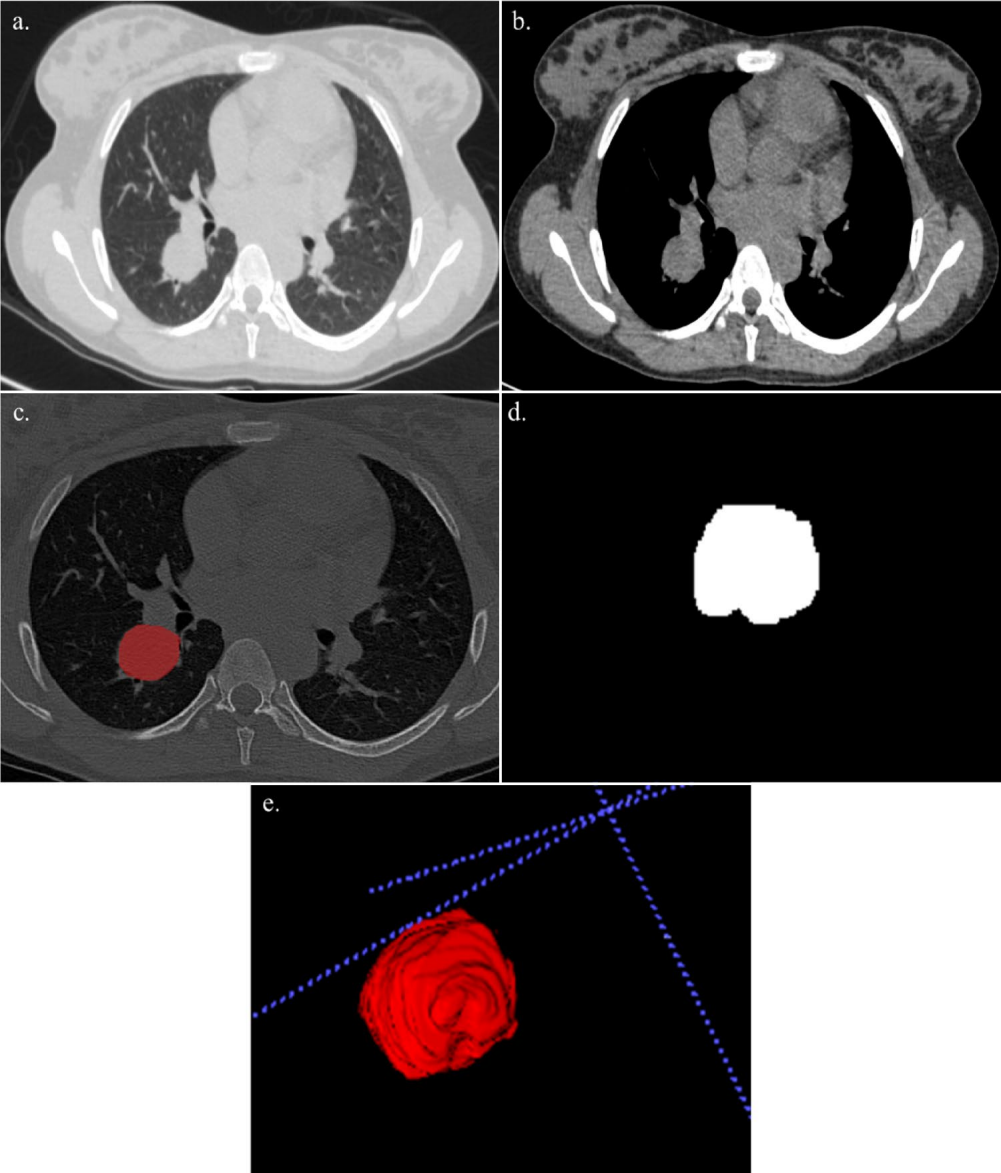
A 21 year-old lady with cough and hemoptysis. HPE: benign carcinoid tumor. (a,b) Axial section of CT thorax in lung window and soft tissue window showing a mass lesion in the posterior segment of the right lower lobe. (c) Creation of ROI for segmentation. (d) 2D segmented mass lesion. (e) 3D volumetric rendering of the mass lesion.
